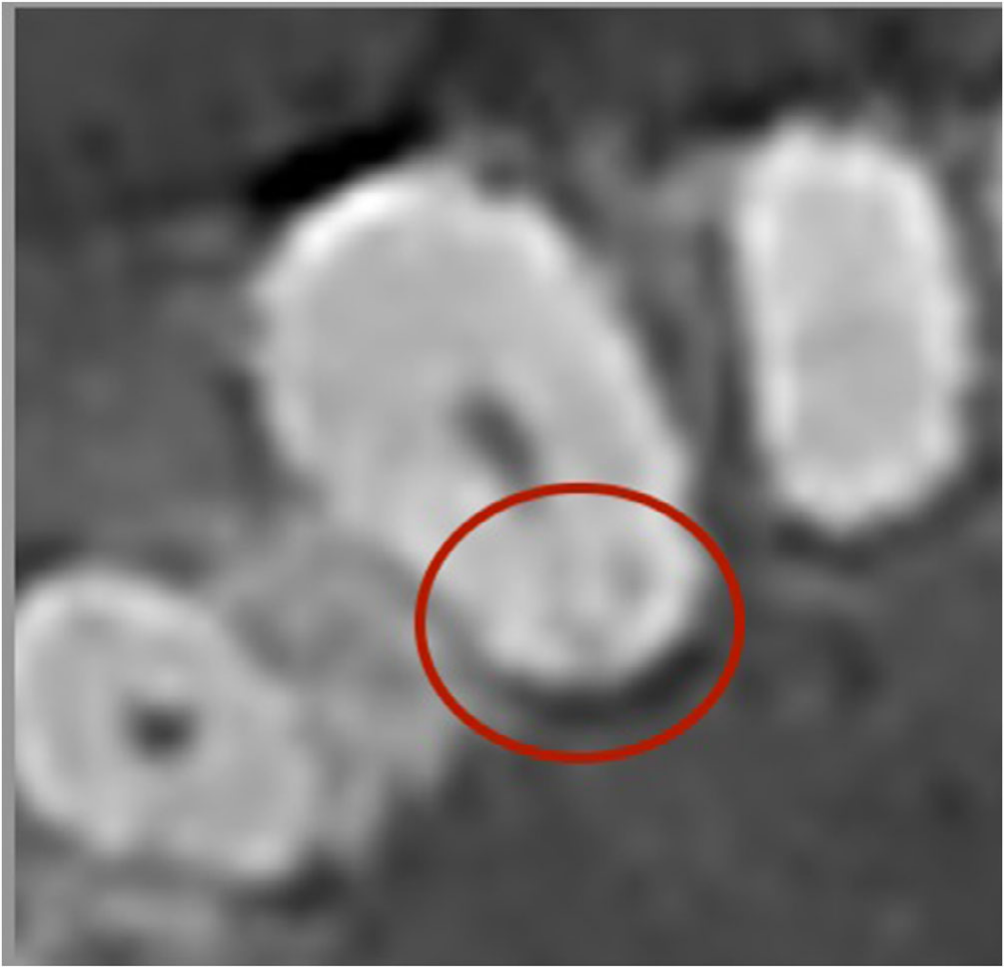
Cone‐beam computed tomography axial image of fractured tooth presenting graphical irregularity.
